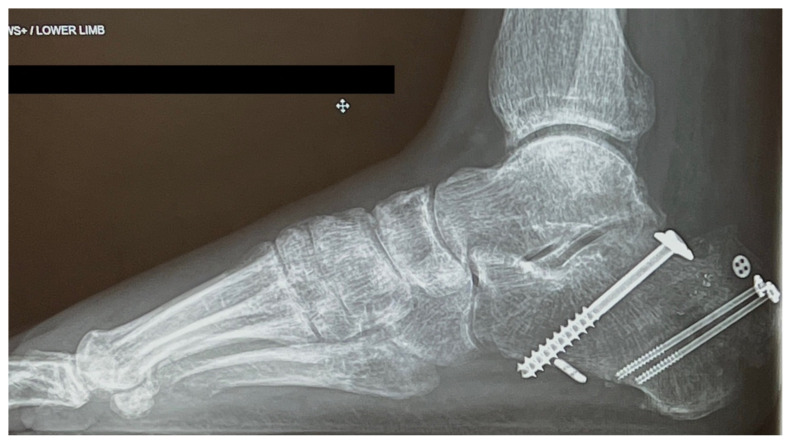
X-Ray 2 months After 3rd Surgery
